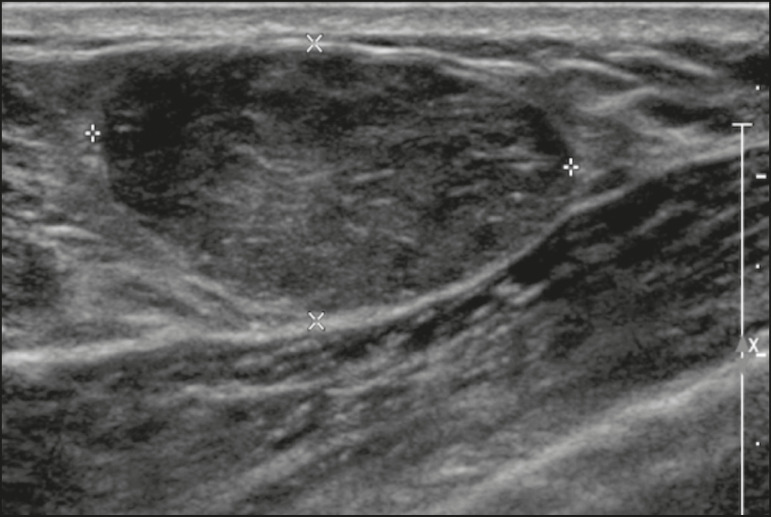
Ultrasound of the right breast showing an oval, hypoechoic, circumscribed mass, with no posterior acoustic features and with orientation parallel to the skin. Core needle biopsy confirmed the diagnosis of lactating adenoma.
